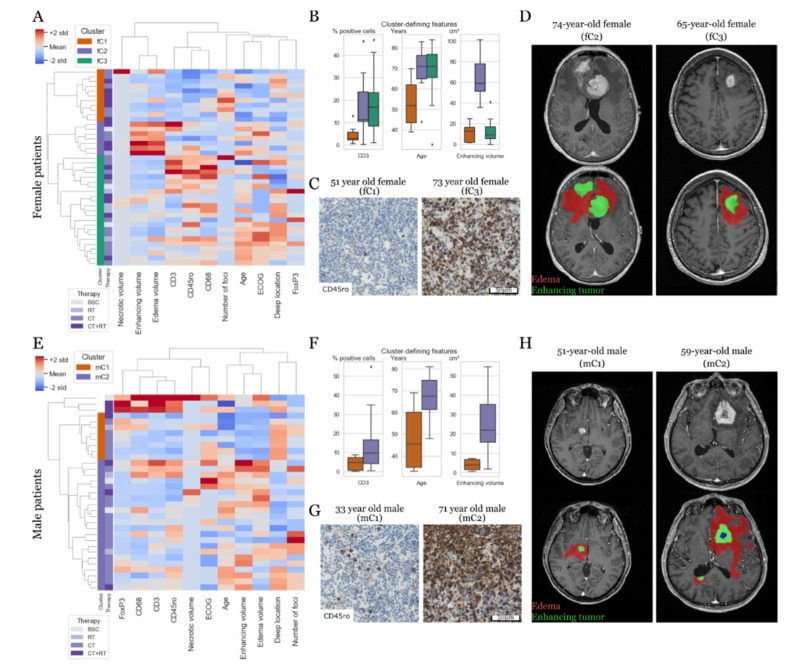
Cluster analysis (Euclidean distance, Ward-linked) based on numerical and ordinal variables. (A–D) Female patients. (E–H) Male patients. (A) Cluster analysis of the female dataset results in three clusters that significantly differ in the combination of features, three of which are depicted in (B), such as immune response (CD3+ TILs), age, and contrast enhancement (all *p* < 0.01, ANOVA). (C) Comparison of the CD45ro+ immune response of two representative female patients. (D) Comparison of the MR imaging features of two representative female patients. (E) Cluster analysis of male patients yields two main clusters. (F) Examples of cluster-defining features include immune response (CD3+ TILs), age, and contrast enhancement (all *p* < 0.01, ANOVA). (G) Comparison of the CD45ro+ immune response of two representative male patients. (H) Comparison of the MR imaging features of two representative male patients.
